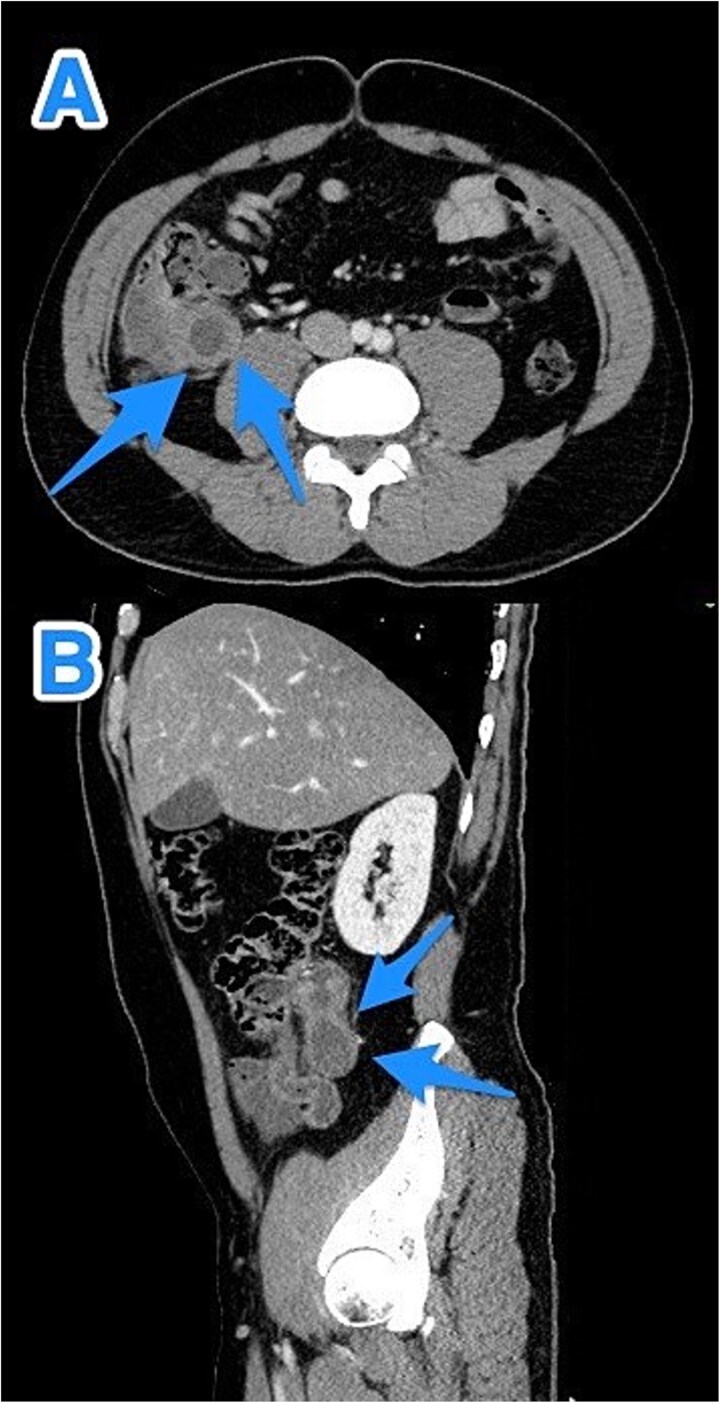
CT abdomen and pelvis with contrast—Axial (A) and sagittal (B) views highlighting a dilated, fluid-filled appendix with a maximum diameter of 2.2 cm and small peripheral calcifications (arrows).
